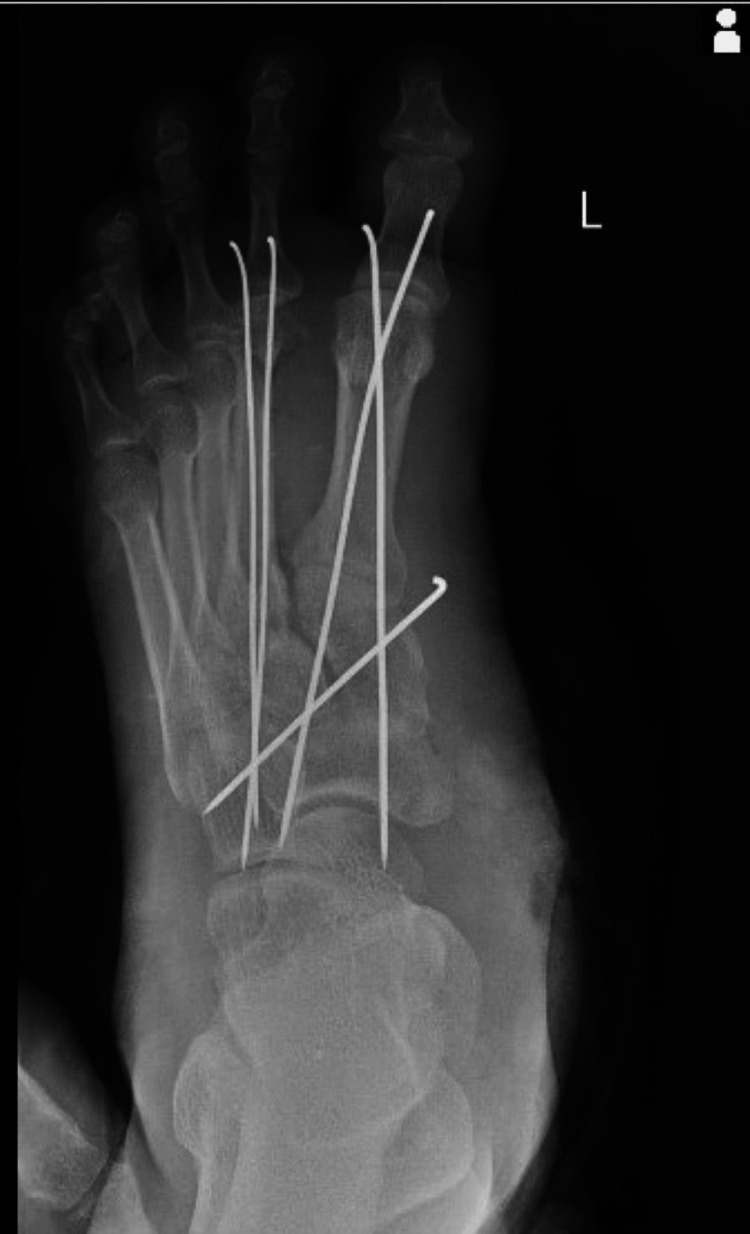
Post-operative X-ray with K-wire fixation
